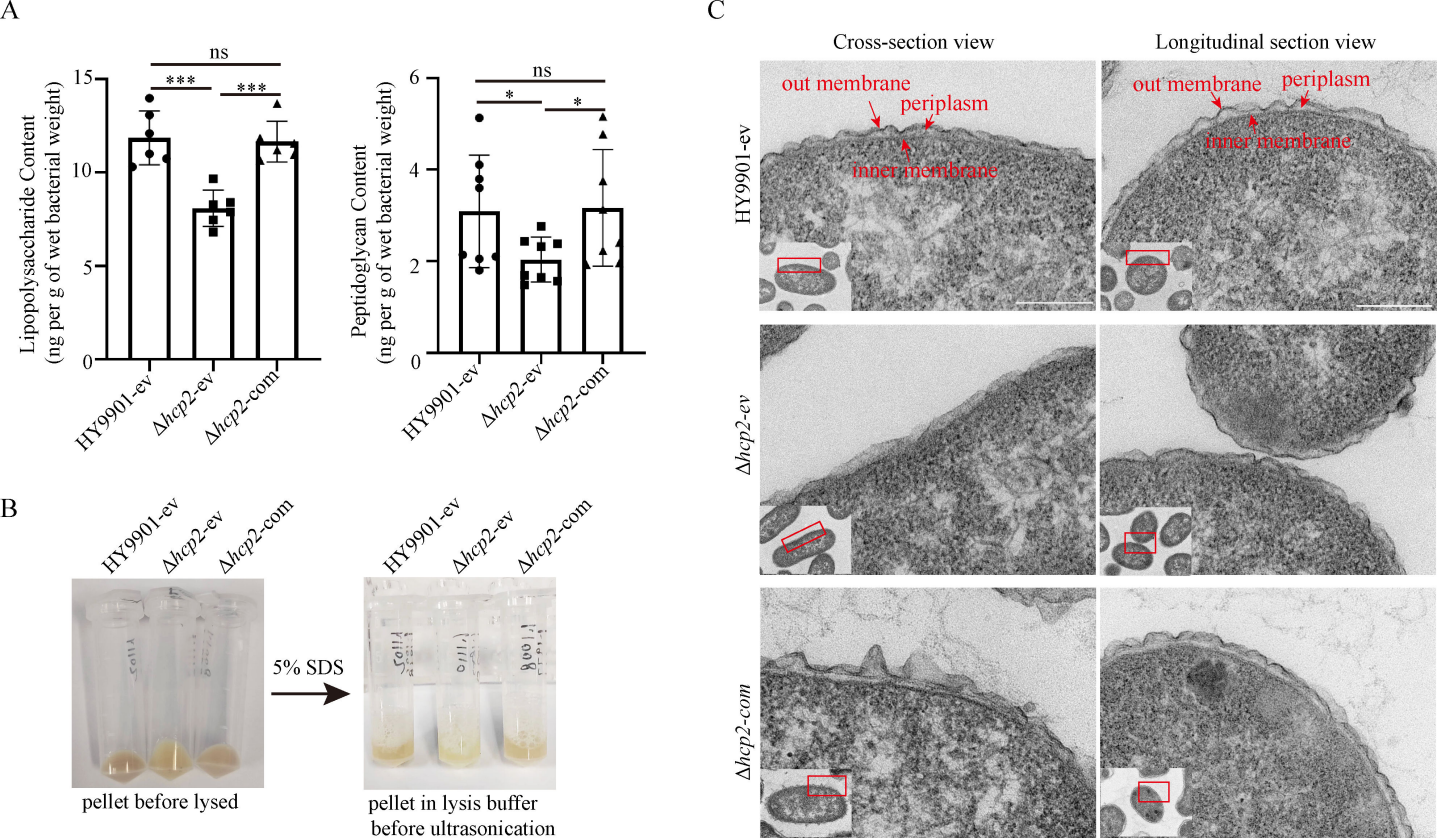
Quantitative analysis of LPS and PGN and ultrastructural observation of the cell wall of *V. alginolyticus.* (A) Quantitative analysis of LPS and PGN in *V. alginolyticus* HY9901, *hcp2* mutant, and *hcp2* complement strains. (B) The pellet state and the solubility of *V. alginolyticus* HY9901-ev, Δ*hcp2*-ev, and Δ*hcp2*-com strains in 5% SDS lysis buffer before ultrasonication. The mutant strain displayed a phenotype of increased susceptibility to lysis, yielding a lysate that was notably more transparent compared with the wildtype and complemented strains. (C) Comparison of cell wall ultrastructure in *V. alginolyticus* HY9901-ev, Δ*hcp2*-ev, and Δ*hcp2*-com strains. The TEM results showed that the *hcp2* mutant strain had a lower electron density of the outer membrane and a more blurred boundary with the periplasm compared with wildtype strain. The phenotype defect caused by the loss of *hcp2* was partially rescued in the complemented strain. HY9901-ev: the wildtype strain carried with pBBR1-MCS1 empty vector; Δ*hcp2-*ev: the Δ*hcp2* strain carried with pBBR1-MCS1 empty vector; Δ*hcp2-*com: the Δ*hcp2* strain carried with pBBR1-MCS1-*hcp2* vector. P* < 0.05, P* < 0.001. ns, no significance.
